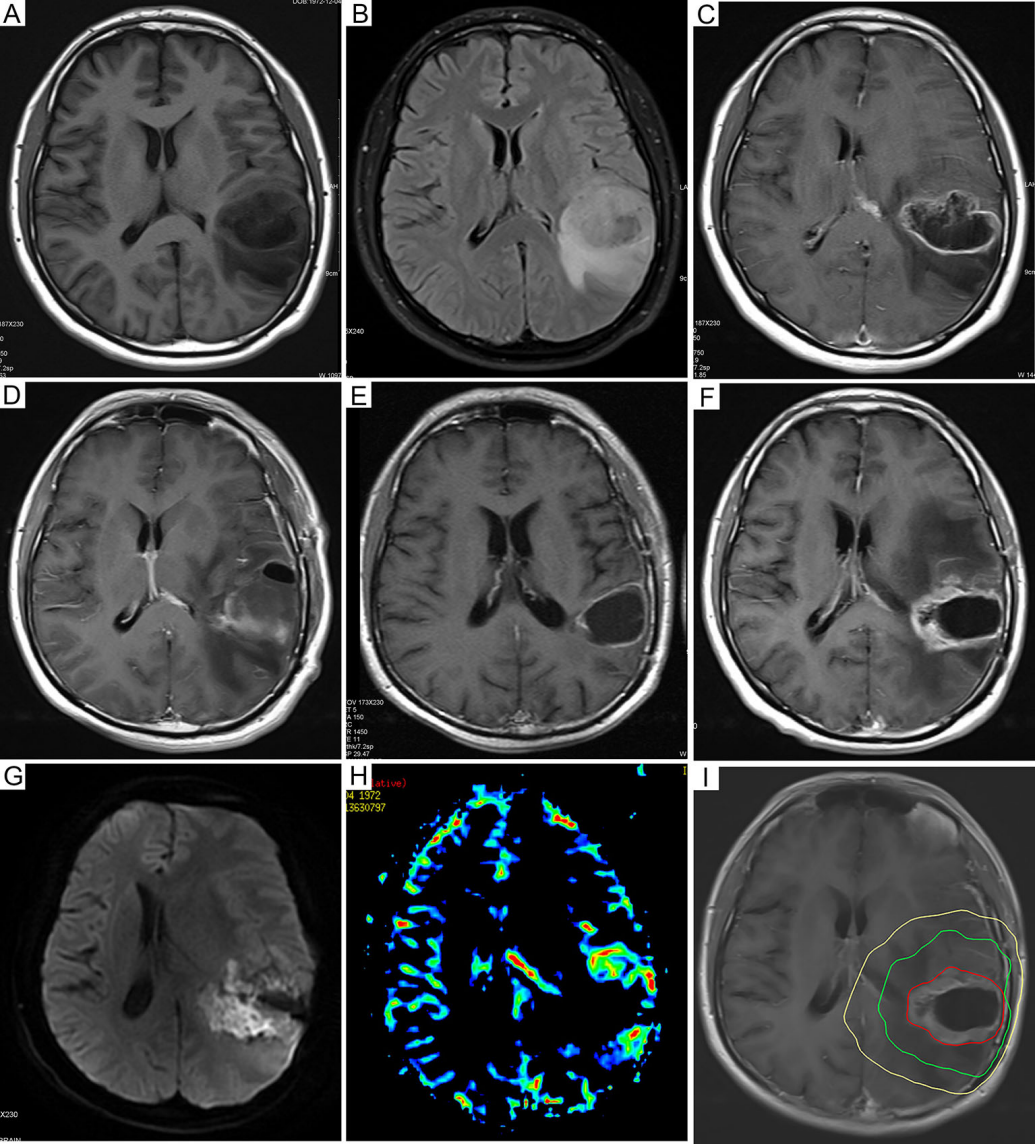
Dynamic follow-up cranial MRI and recurrence mode images of representative GBM patients. (A) Preoperative axial plain T1-weighted images and (B) axial fluid-attenuated inversion recovery images before resection. (C) Preoperative axial enhanced T1-weighted images showed that the tumor was in the left temporoparietal lobe. (D) Axial enhanced T1-weighted images 72 hours after resection. (E) The axial enhanced T1-weighted follow-up images 6 months after the operation showed new enhanced lesions at the level of the primary lesions. (F) Six months after resection, the axial enhanced T1-weighted follow-up images showed new enhanced lesions at the level of the primary lesions. (G) Further examination of DWI showed that local diffusion was limited, as well as high signal intensity. (H) In addition, magnetic resonance PWI showed nodular hyperperfusion around the lesion. The axial enhanced T1-weighted images of (I) were fused with the CT images of radiotherapy planning, indicating central recurrence. The yellow line, the green line, and the red line represent the isodose lines of 54 Gy, 60 Gy, and the volume of tumor recurrence, respectively. MRI, magnetic resonance imaging; GBM, glioblastoma; DWI, diffusion weighted imaging; PWI, perfusion weighted imaging.
